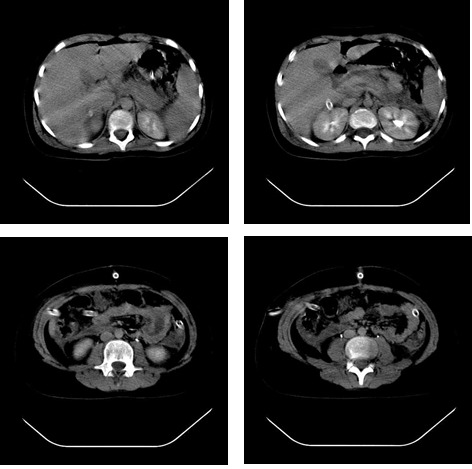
Important aspects of CT scan at admission in our unit
